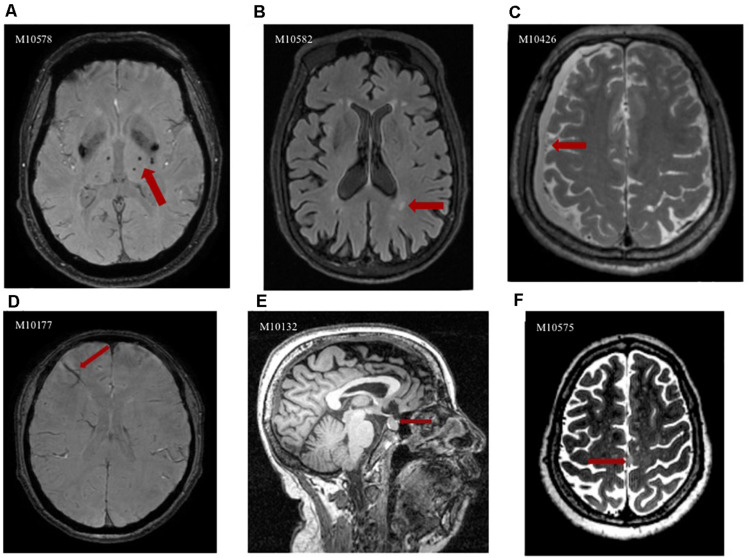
Abnormalities incidentally discovered in the Shanghai Changfeng study IFs could be observed on different images; here are some examples; the imaging features of the abnormalities are indicated with red arrows in each case. (A) Axial SWI image clearly depicts CMBs in the thalamus as hypointense areas; (B) Axial FLAIR image shows hyperintense areas in the deep white matter, representing WMH; (C) Axial T2-weighted image depicts crescent-shaped lesion that is isointense relative to the cerebrospinal fluid, findings that indicate chronic subdural hematoma; (D) Axial SWI image reveals venous malformation in the frontal lobe as hypointense atypically shaped areas; (E) Sagittal T1-weighted image demonstrates well-defined rounded foci that are isointense relative to gray matter in the sella turcica, representing pituitary adenoma; (F) Axial T2-weighted image shows meningioma as a hyperintense extra-axial mass that associated with the falx cerebri.
